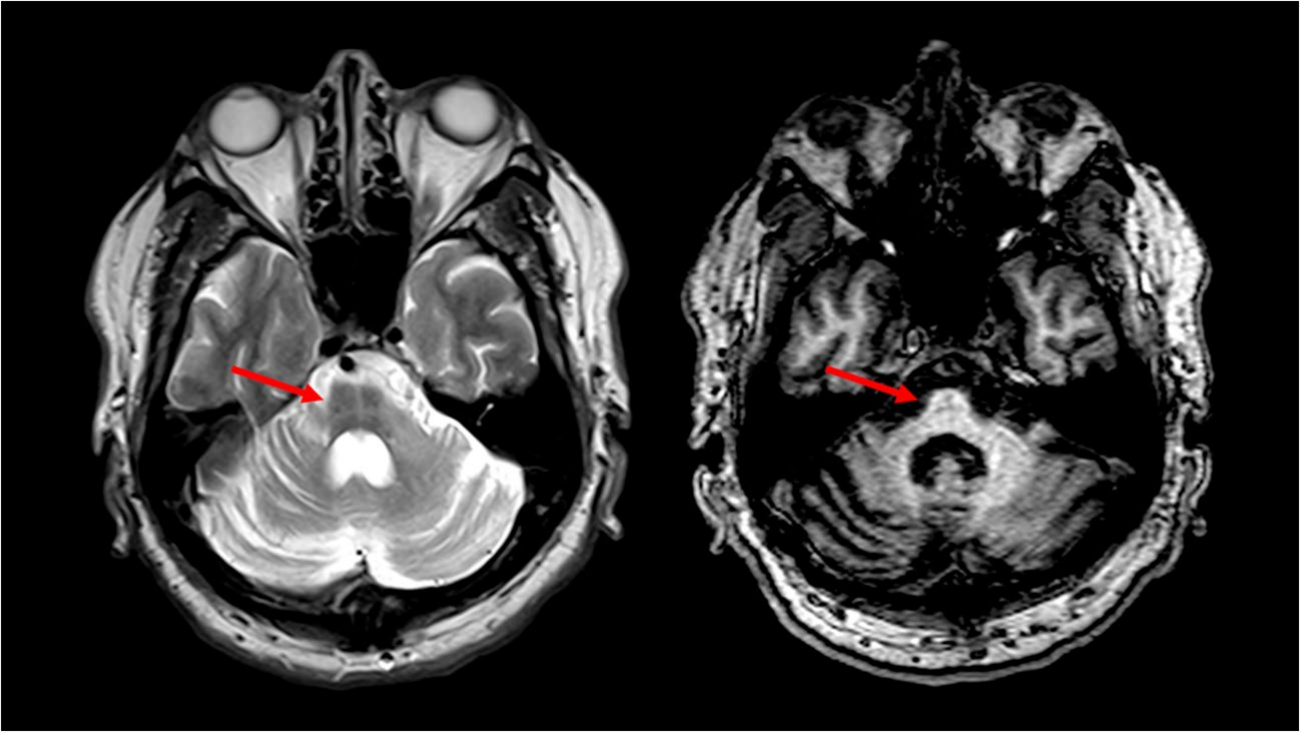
An example of a “Hot Cross Bun” sign. Axial T2-weighted (*left*) and axial multiplanar reconstruction of a T1-weighted volumetric sequence (*right*) of a 59-year-old male Spinocerebellar Ataxia Type 2 patient showing an hyperintensity of transverse pontine fibers and median pontine raphe (*red arrows in left*), also visible as a T1-weighted mild hypointensity (*red arrows in right*), representing the so-called “Hot Cross Bun” sign
